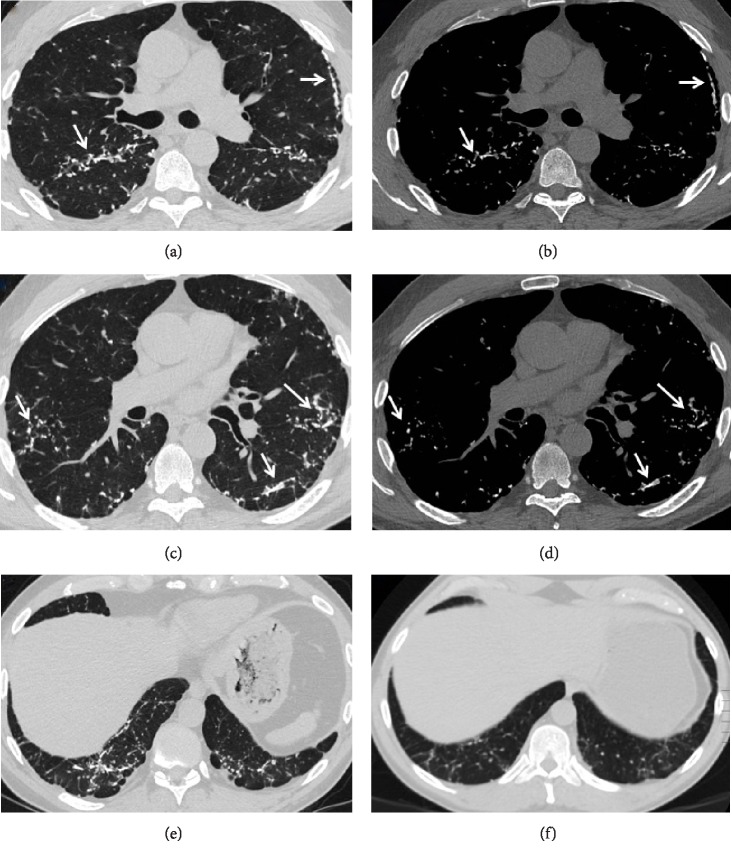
Axial chest CT images using lung (a), (c) and bone (b), (d) windows show bilateral branching dense nodular opacities (arrows) with mild associated reticulation. Some of the nodule are high in attenuation and almost iso-dense to ribs on bone windows. Axial images using lung widow (e), (f) at the level of lung bases were obtained 5 years apart and show evidence of progression.
